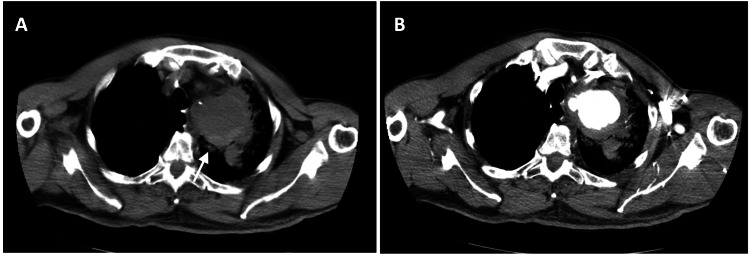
Chest CT angiography on the axial plane: (A) non-contrast acquisition showing hyperdensity suggestive of recent hemorrhage with periaortic and intrapulmonary extension (white arrow); (B) contrast-enhanced axial image revealing intense enhancement in the previously hyperdense areas. CT, computed tomography
